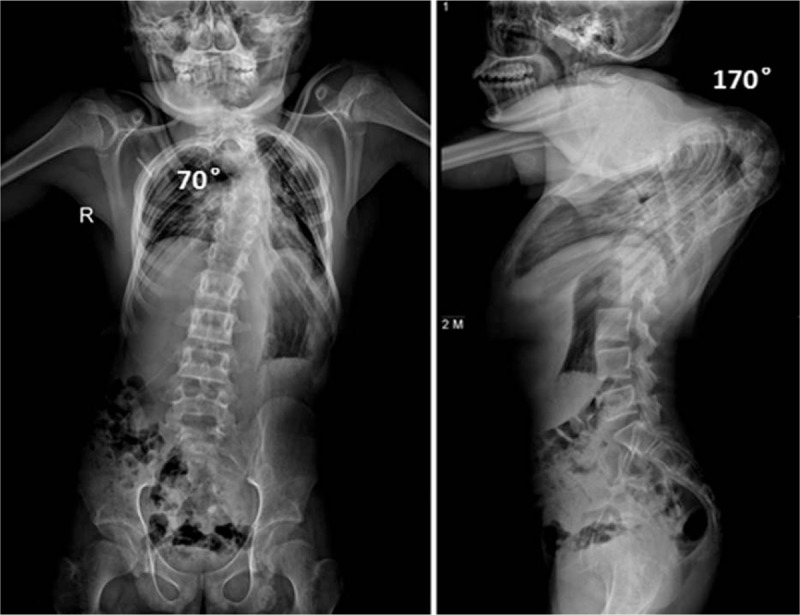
Preoperative scoliosis radiography showed coronal and thoracic kyphosis Cobb's angles of 70° and 170°, respectively.
